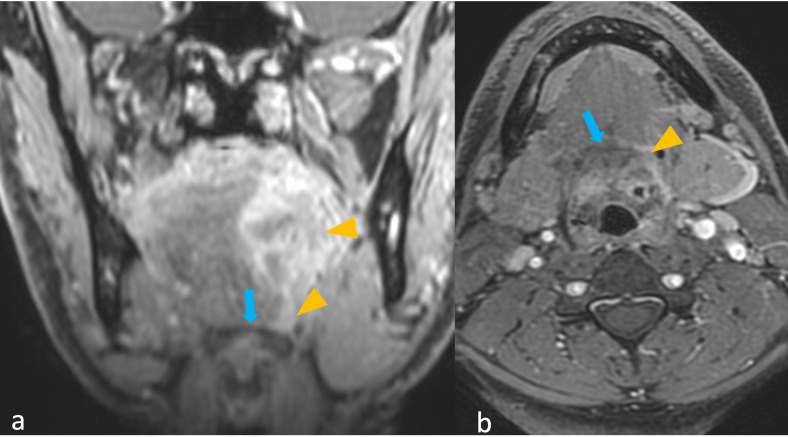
(a, b) MRI images of oral tongue squamous cell carcinoma with tumor reaching the hyoid bone. Coronal contrast-enhanced (CE) fast spoiled gradient echo (FSPGR) image (a) and axial CE FSPGR image (b) show tumor (arrowheads) extending to the hyoid bone (arrows).
